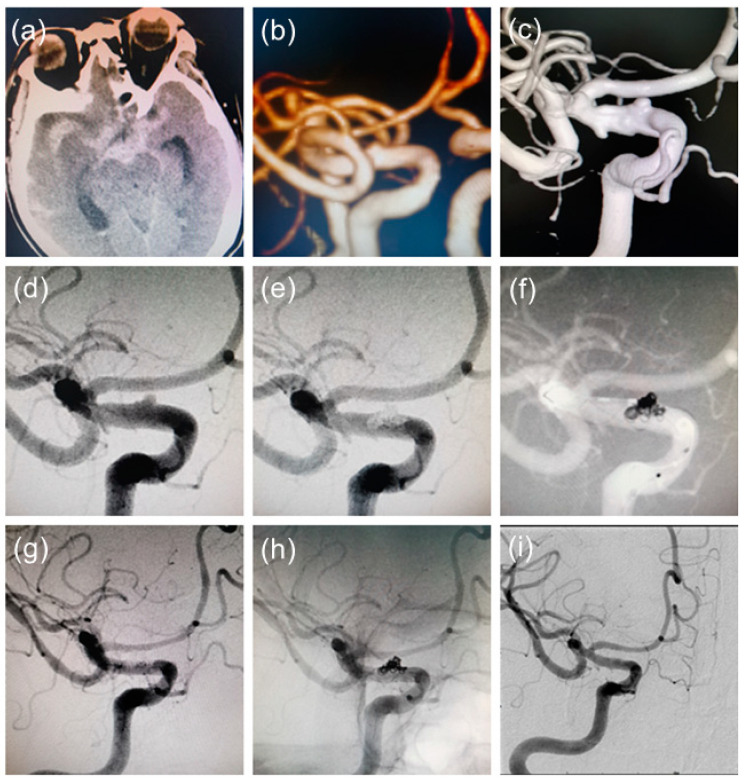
56-year-old female presented with sudden unconsciousness, and an axial CT head showed a subarachnoid hemorrhage (a). A pretreatment CT angiogram exhibited an aneurysm in the clinoid segment of the left intracranial artery (b). DSA examination and 3D reconstruction cleared the ruptured blood blister-like aneurysm (treatment angle) (c,d). During the process, the patient underwent FDD-assisted coiling via a semi-deploying method (Tubridge 4.0 × 20 mm). After treatment, the aneurysm was completely occluded (e,f). Follow-up angiograms at 3 months (g,h) and 6 months post-procedure showed complete resolution of the aneurysm with remodeling of the parent vessel (i).
